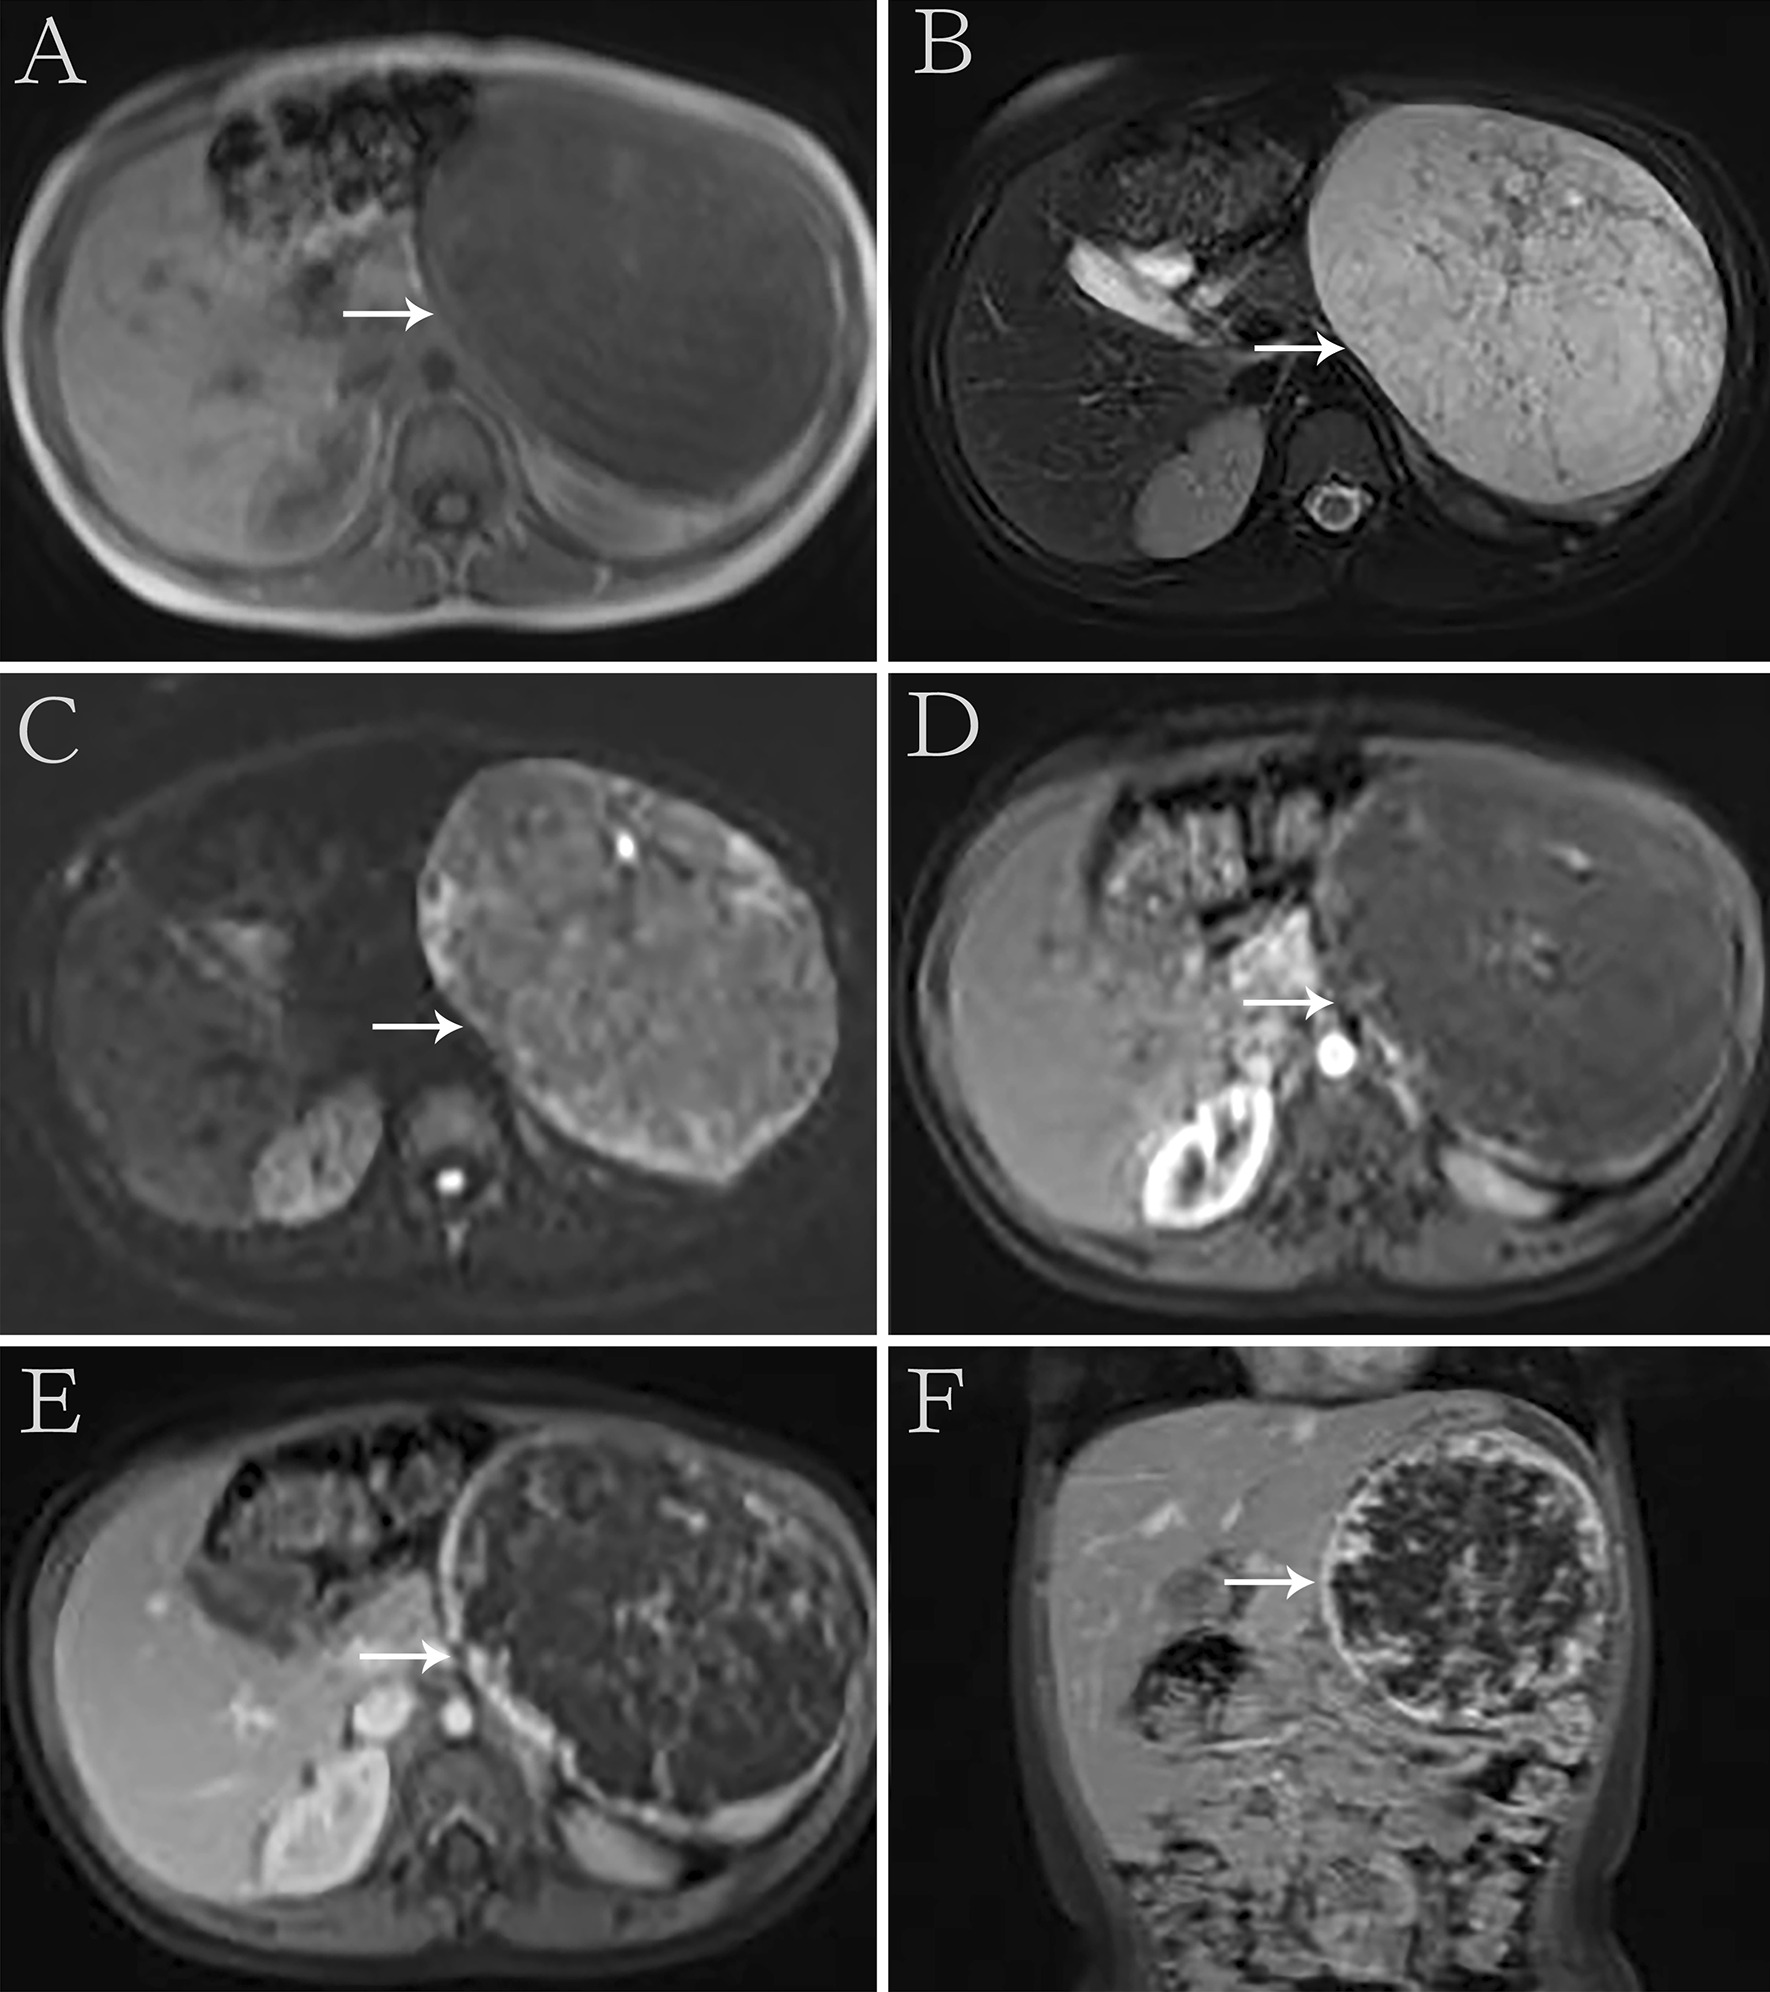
Magnetic resonance imaging shows the mass was globally hypointense on T1 weighted images, with some foci of increased signal intensity (A). Mildly heterogeneous, with predominantly high signal and some internal elongated (or linear) hypointense structures are shown on the T2-weighted images (B). Diffusion weighted images (b = 1000 s/mm^2^) demonstrated a heterogeneous pattern of increased diffusion, more prominent in the periphery (C). Multiple dilated and tortuous nutrient arteries were observed in the enhanced scanning arterial phase (D). Multiple foci of enhancement, with flaky appearances were observed in the portal phase (E). In the delayed period, continuous enhancement is seen in the lesions, with obvious enhancement at the edge (F)
